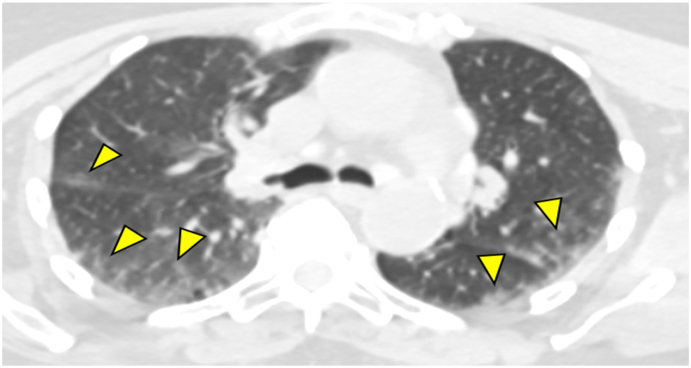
Axial CT of the chest (lung window) shows multiple bilateral subpleural and peripheral ground glass opacifications and atelectatic changes (yellow arrow heads) suggestive of COVID-19 pneumonia. (For interpretation of the references to colour in this figure legend, the reader is referred to the Web version of this article.)
